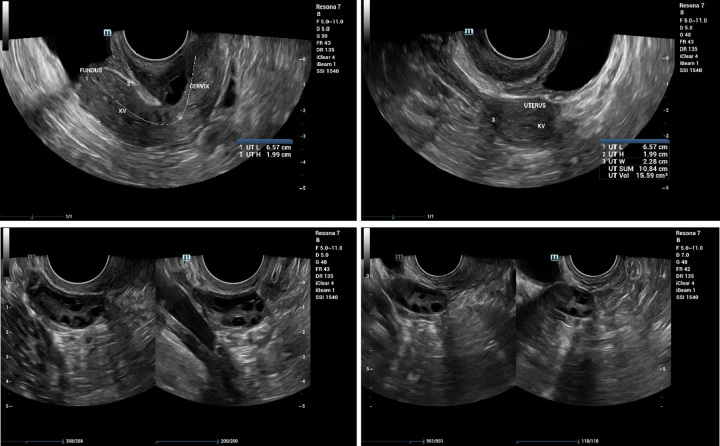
Transvaginal ultrasound.
